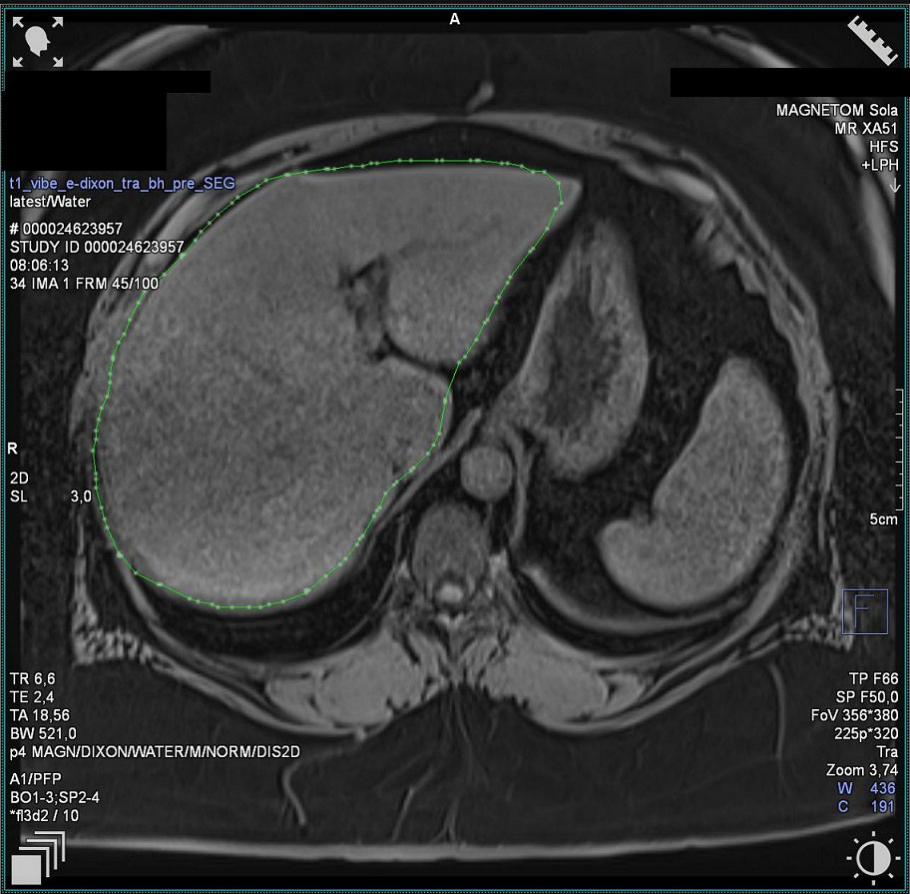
Case 2- Liver MRI image 1 (19.3% of fat content, N<5%).
